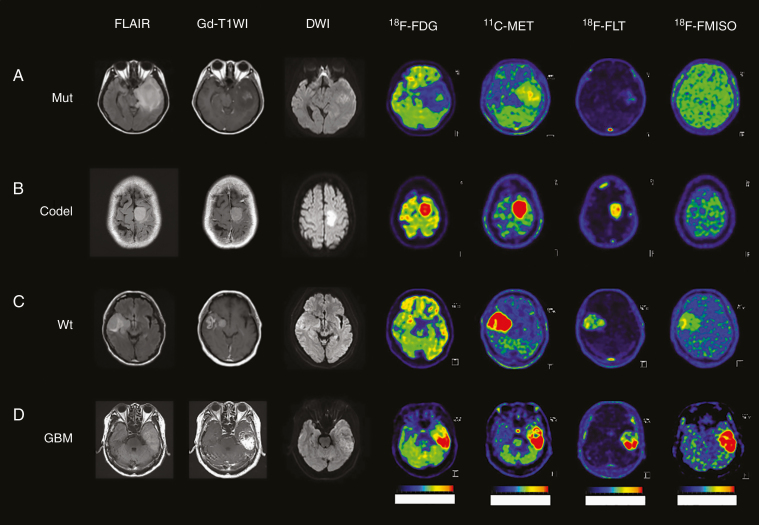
MRI (FLAIR, Gd-T1WI, and DWI) and PET images (^18^F-FDG, ^11^C-MET, ^18^F-FLT, and ^18^F-FMISO) in representative glioma patients. (A) A 29-year-old female patient in Mut subtype with the accumulation of ^11^C-MET and ^18^F-FLT. (B) A 38-year-old male patient in Codel subtype with high accumulation of ^11^C-MET, and slight accumulation of ^18^F-FLT. (C) A 58-year-old male patient in Wt subtype with the accumulation of ^11^C-MET and higher accumulation of ^18^F-FLT and ^18^F-FMISO than Mut. (D) A 69-year-old male patient with GBM with the highest accumulation of ^11^C-MET, ^18^F-FLT, and ^18^F-FMISO.
